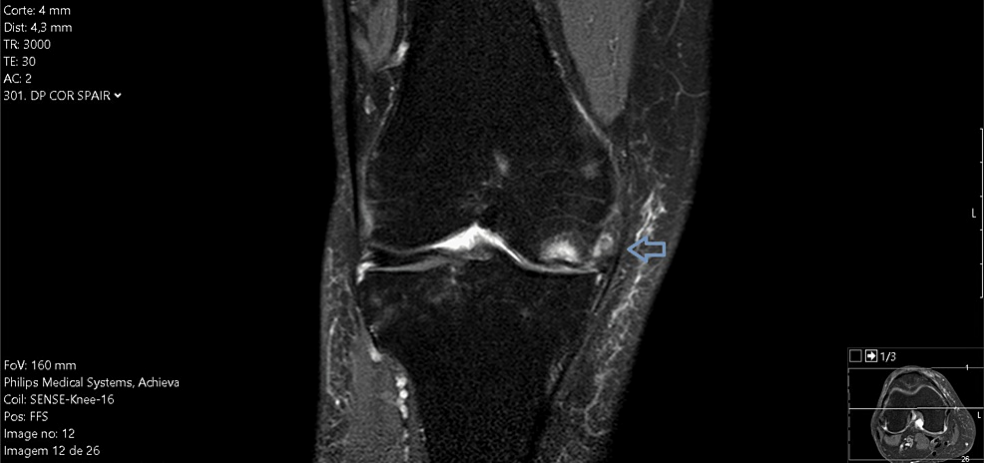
MRI of the right knee in the coronal plane (re-evaluation).
